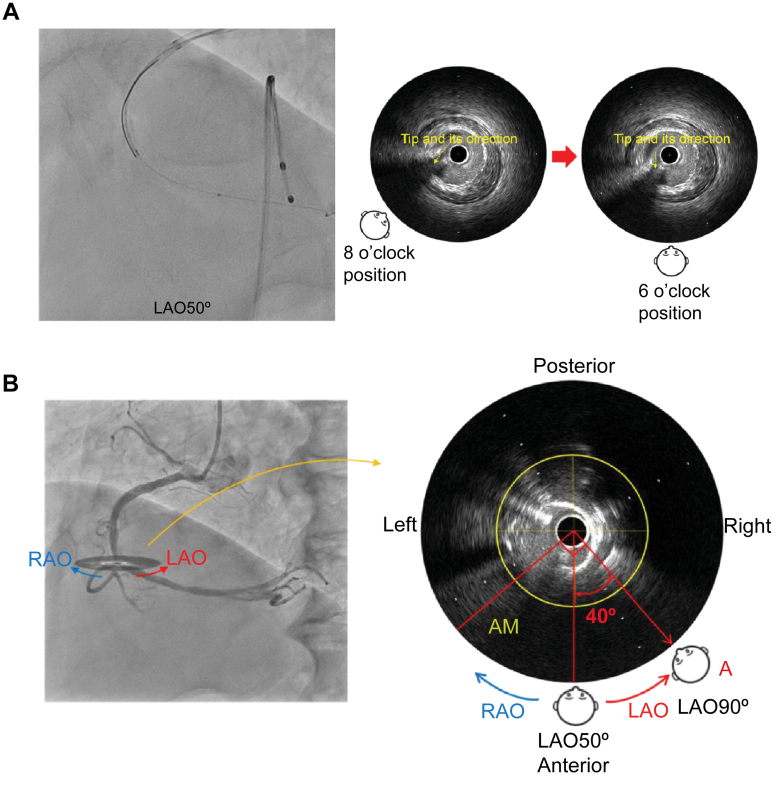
Calculation of the Optimal Angiographic View Using IVUS (A) To determine the angiographic view on intravascular ultrasound (IVUS), the tip of the guidewire is directed toward the detector, that is, toward the operator. By using the tip detection method to visualize the tip direction on IVUS, the operator's viewpoint is determined and set at the 6 o'clock direction. This alignment allows the IVUS image to be superimposed on the angiographic image. (B) The angle relative to the position perpendicular to the acute marginal (AM) branch on the IVUS image is 40° counterclockwise from the 6 o'clock direction. Therefore, the optimal angiographic view for clearly distinguishing the bifurcation is left anterior oblique (LAO) at 90°. RAO = right anterior oblique.
